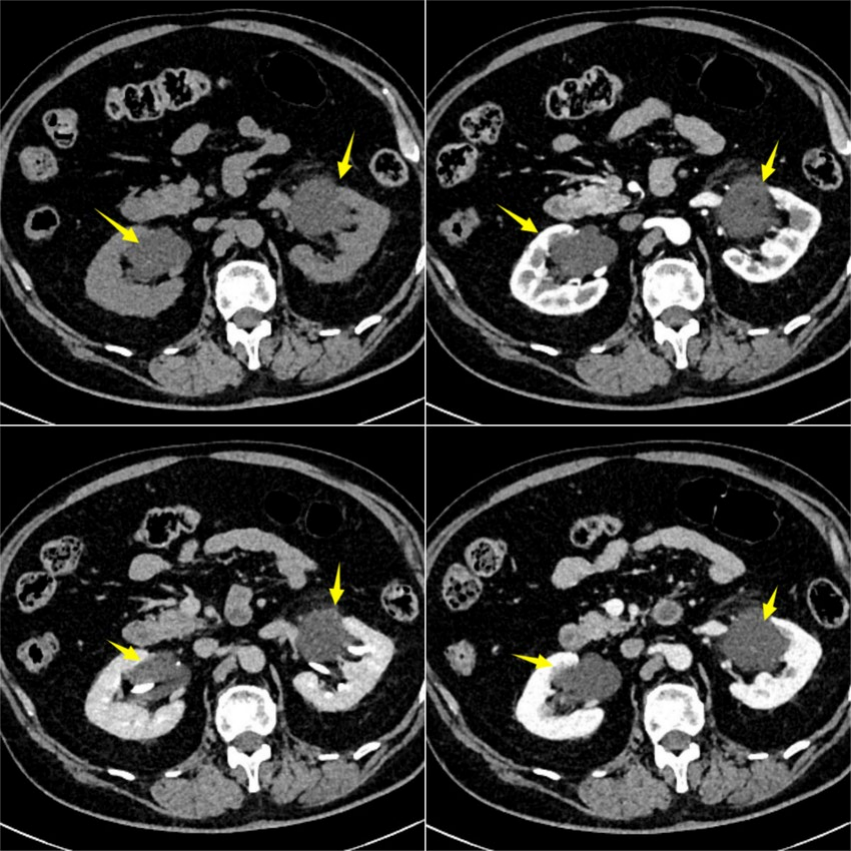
Contrast-enhanced CT showing cystic low-density lesions in bilateral renal pelves (as indicated by arrows). No obvious enhancement is observed on post-contrast images. On delayed-phase imaging, the renal pelves are opacified with contrast medium, and the calyces remain uncompressed bilaterally.
